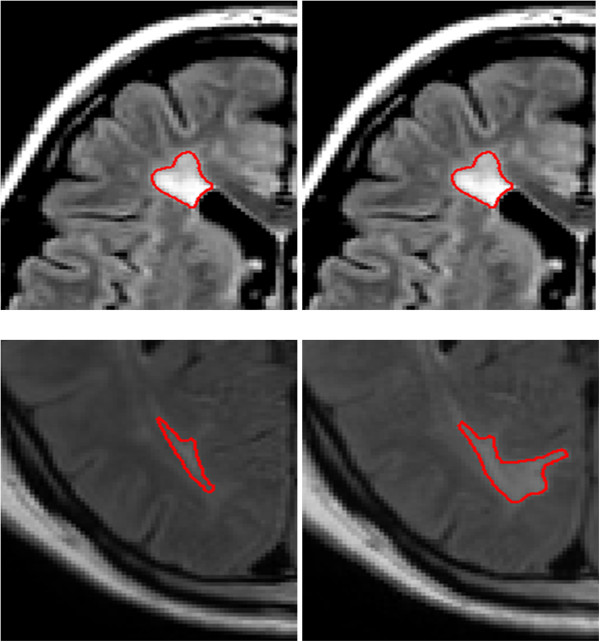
The left images are from a FLAIR MRI baseline scan of a patient with MS, and the right images are the matching planes at 3 month follow-up. MACC was used to propagate ROIs drawn of the lesions on the baseline scan to automatically create the ROIs on the follow-up scan. Since MACC creates the lesion ROIs based on the underlying image topography (in this case the follow-up scan), the automatically drawn follow-up ROIs accurately reflect both the changes in the size and shape of the lesion.
